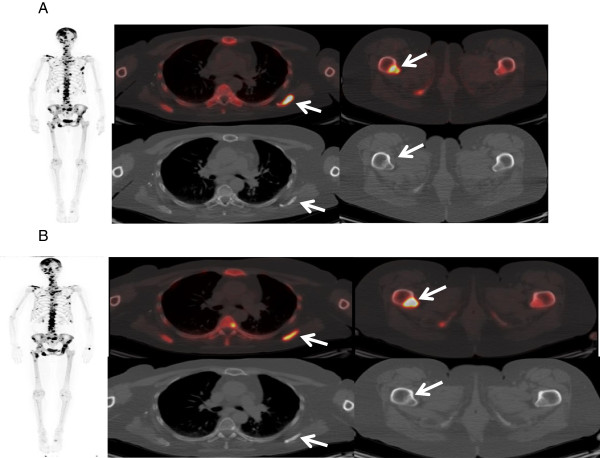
Imaging study with ^18^Na-F Bone PET/CT. A: ^18^NA-F bone PET/CT imaging studies at baseline, before administration of radium-223 (Ra-223) dichloride, B: ^8^NA-F bone PET/CT imaging studies after 2 treatments with Ra-223 dichloride but before the third treatment.
